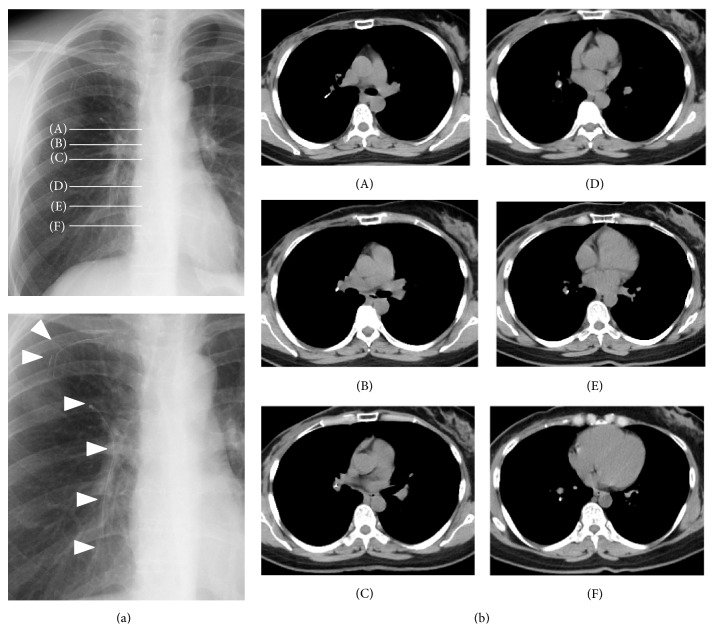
(a) Chest radiographs showing catheter dislodgement due to catheter pinch-off syndrome. Alphabets indicate the slice level of computed tomography. White arrowheads indicate the dislodged catheter and the residual part of the implanted central venous port. (b) Computed tomography images. The dislodged catheter is stuck to the upper and lower pulmonary artery branches.
